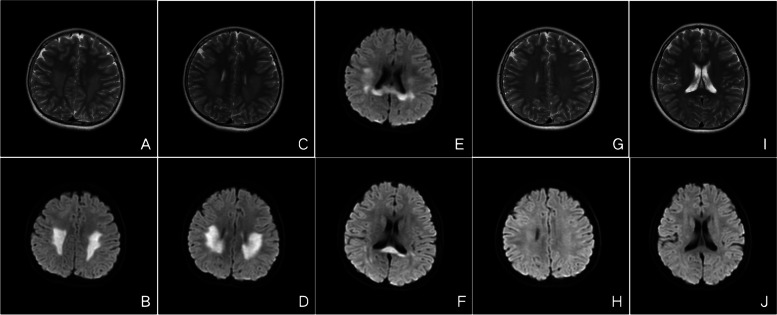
Cranial MRI of case 2 in 2015. Initial cranial MRI showed lesions in the entire corpus callosum and diffuse white matter on T2-weighted images (A,C) and DWI (B,D-F). Follow-up MRI showed resolution of the lesions on T2 (G,I) and DWI (H,J) after seven weeks
